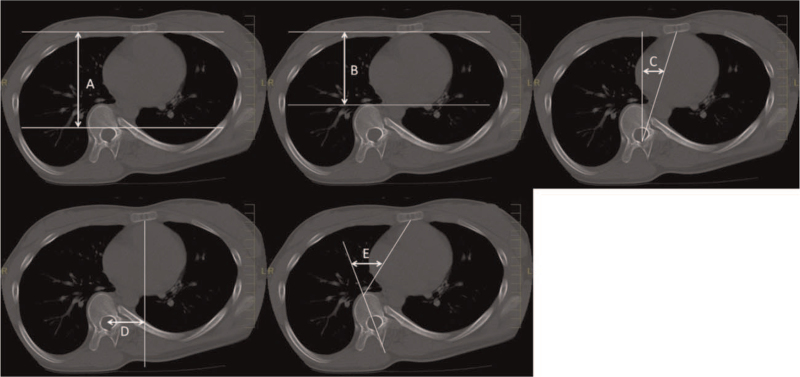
Computed tomography measurement of the thoracic cage parameters at the level of the apical thoracic vertebra. The sagittal diameter, A, is the distance between the posterior midpoint of the sternum and the anterior point of the foramen along the anteroposterior plane. The sternovertebral distance, B, is the distance between the posterior midpoint of the sternum and the anterior point of the apical vertebra along the anteroposterior plane. The midline deviation, C, is the angle between the anteroposterior plane and the line through the posterior aspect of the foramen and the anterior midpoint of the sternum. The vertebral translation, D, is the distance between the anteroposterior planes through the center of the foramen and bisecting the sternum. The thoracic rotation, E, is the angle between the plane bisecting the sternum and the anterior aspect of the vertebral body and the plane bisecting the vertebrae.
